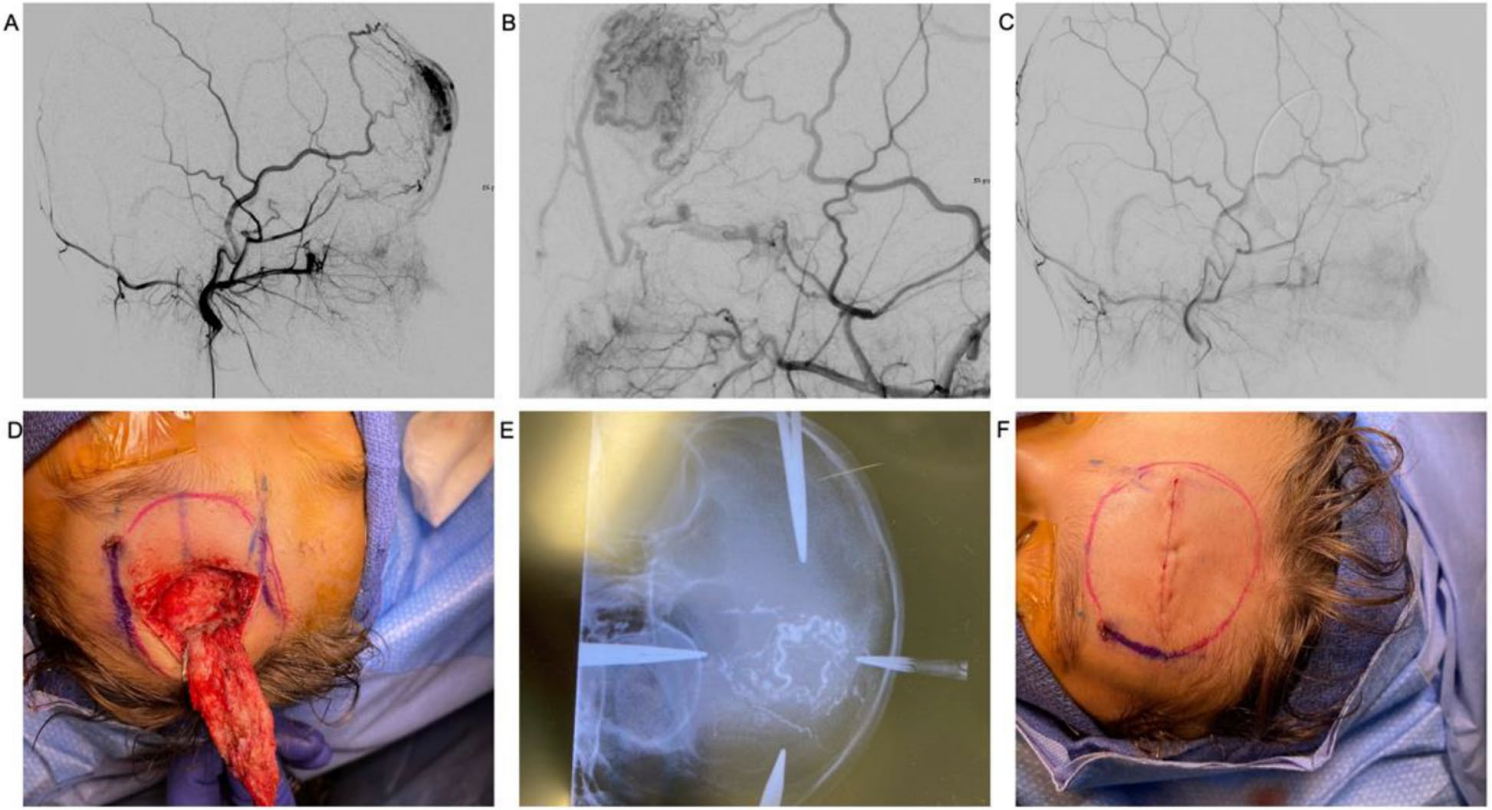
Left forehead AVM with overlying skin changes. A An angiogram via the external carotid artery showing an arteriovenous malformation centered over the left forehead. B Magnified view showing multiple arterial pedicles feeding the AVM. C Angiogram post-embolization with N-BCA via a percutaneous approach. D Excision of AVM. E Intraoperative radiograph performed for nidus localization. F Primary closure of the excision site
